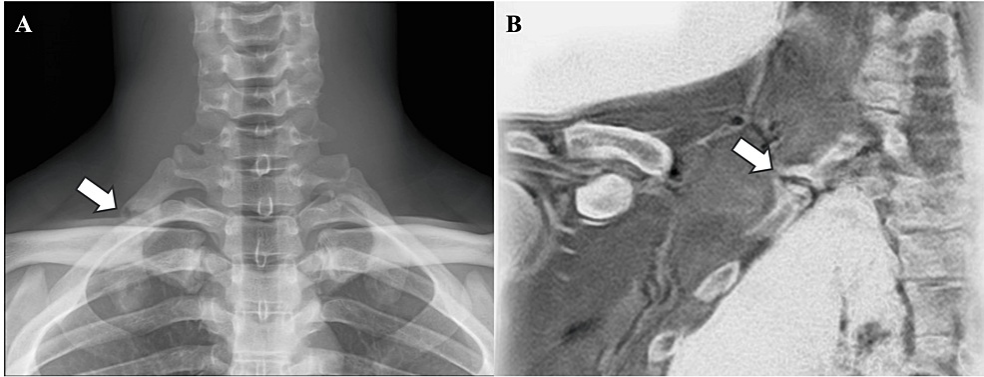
Image findings of the first rib stress fracture The nonunion of the first rib stress fracture was confirmed by radiograph (A) and bone magnetic resonance imaging (B). The arrow indicates a discontinuity in the first rib.
